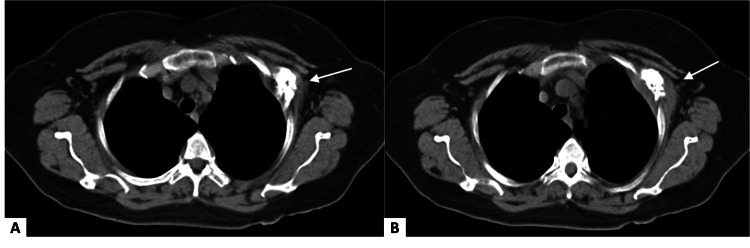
Chest CT scan Axial CT slices show extensive destruction involving the anterolateral portion of the left fourth costal arch, associated with a soft tissue mass (A, B).
